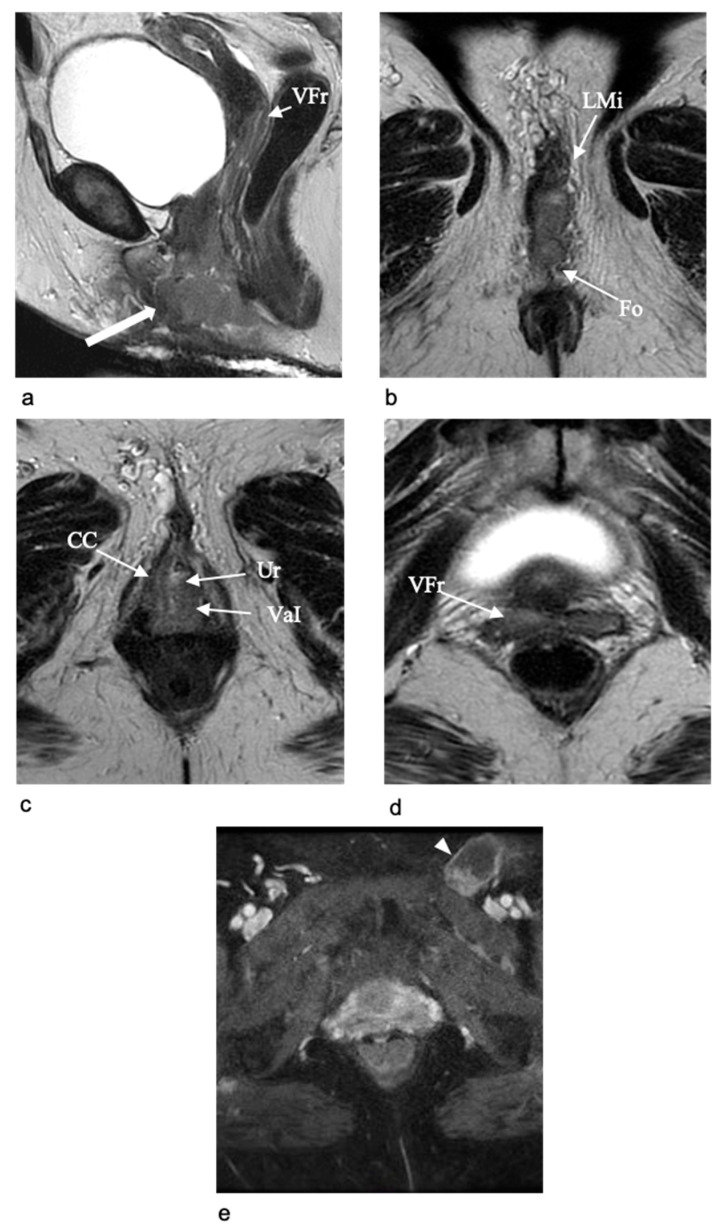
Stage IVA, in an 88-year-old woman with vulvar carcinoma. Sagittal (a) and axial T2-W MR images at the level of perineum (b), of vestibule (c) and of pubic symphysis (d) show a big vulvar mass (arrow) located mainly on the left vulvar side involving the labia minora (LMi) and the fourchette region (Fo) posteriorly. The tumor also infiltrates the clitoris crura (CC) and the vestibule, including the urethral meatus (Ur) and the vaginal introitus (VaI) and extends cranially to the upper vagina into the fornices (VFr). Axial contrast-enhanced T1-W MR image (e) shows an enlarged and necrotic left inguinal lymph node (arrowheads).
